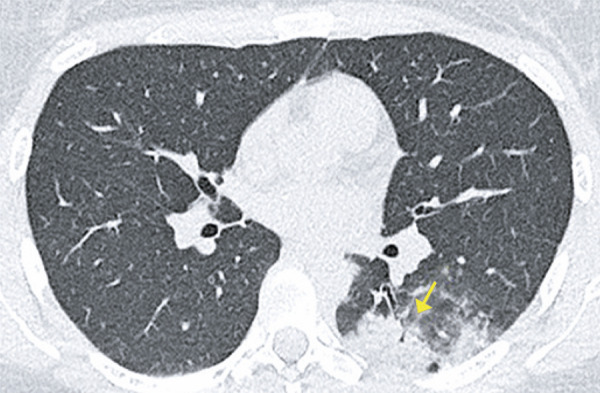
A 28-year-old patient with COVID-19, presenting cough for 15 days. Computed tomography showing peripheral consolidation in the left lower lobe
